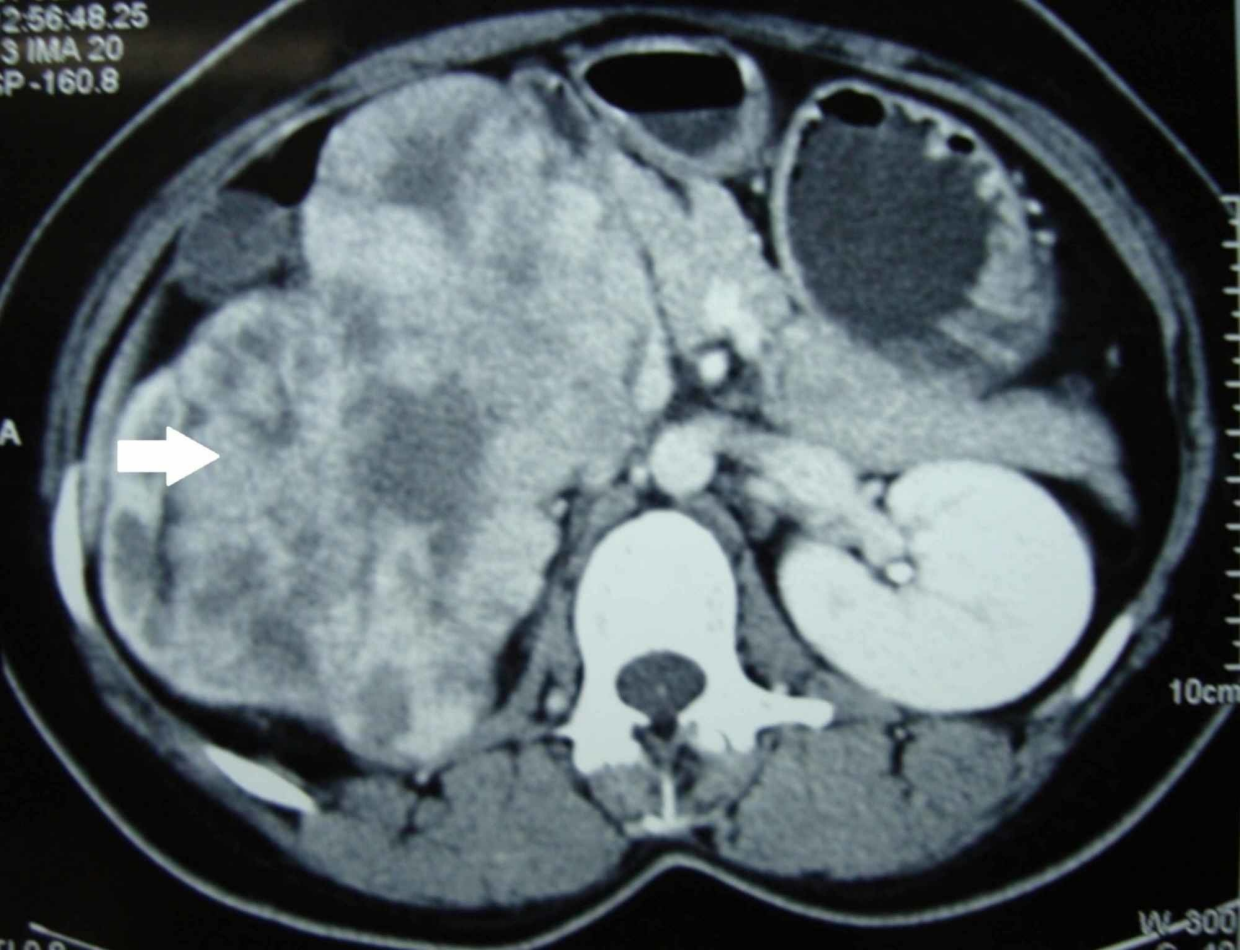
Contrast-enhanced computed tomogram showing the enhancing right renal mass (arrow)
